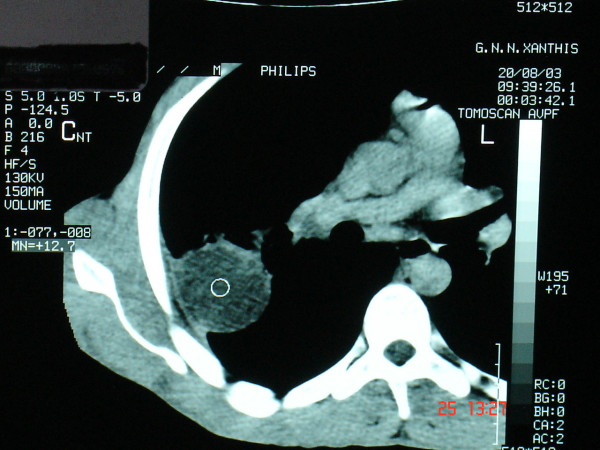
Computed tomography revealed a right lower lobe soft tissue density mass.
